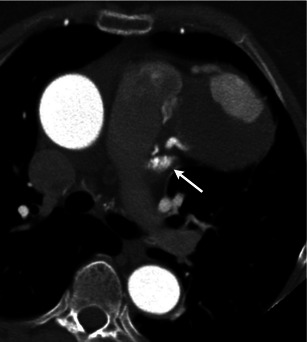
Contrast-enhanced axial CT image in systemic arterial phase demonstrates contrast blush within the pulmonary trunk emanating from a tubular enhancing structure along the left anterior descending coronary artery. Communication is noted between this and the pulmonary artery, suggesting a coronary to pulmonary artery fistula (*arrow*)
